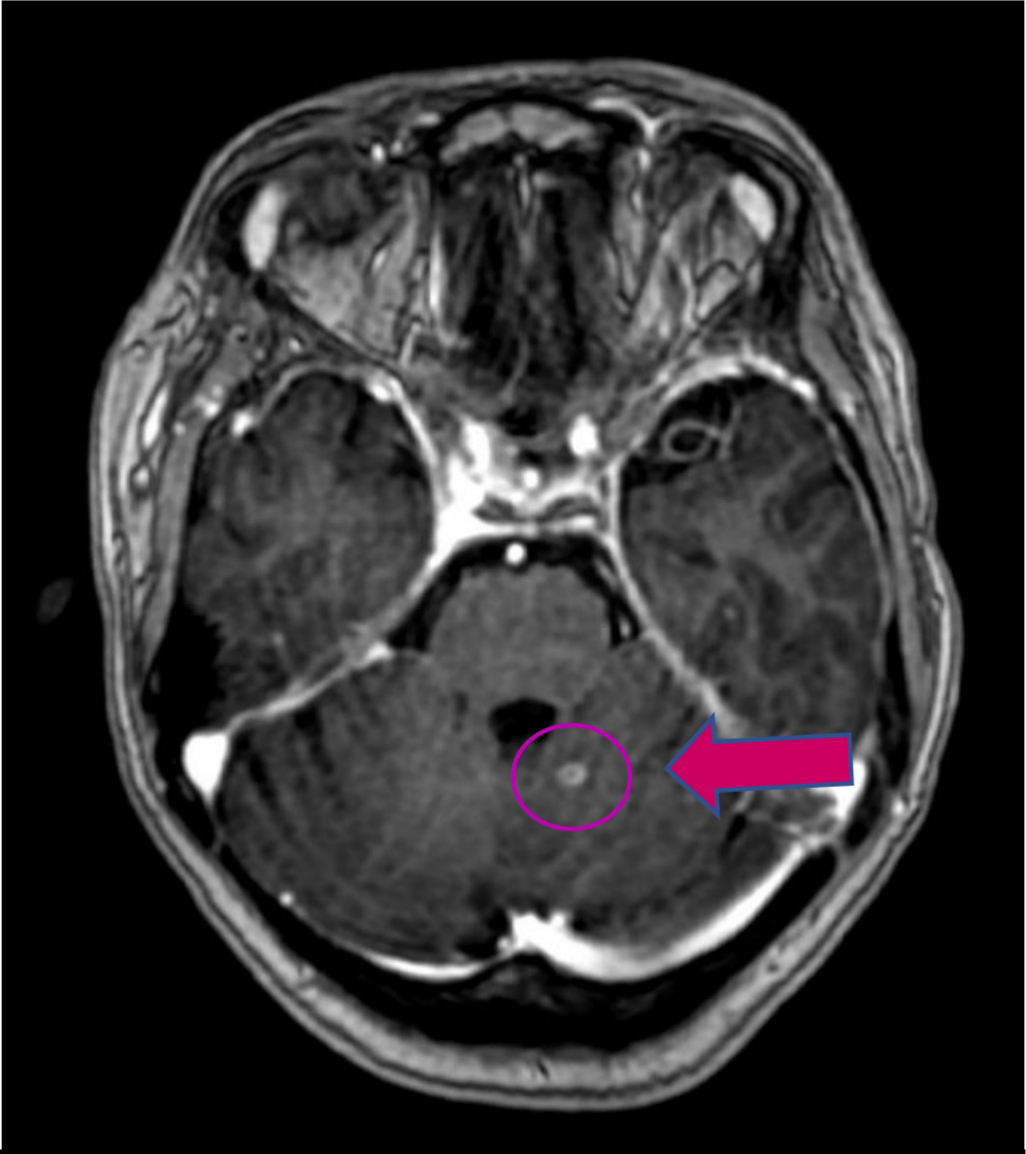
Red arrow showing brain enhancing lesion in MRI.
